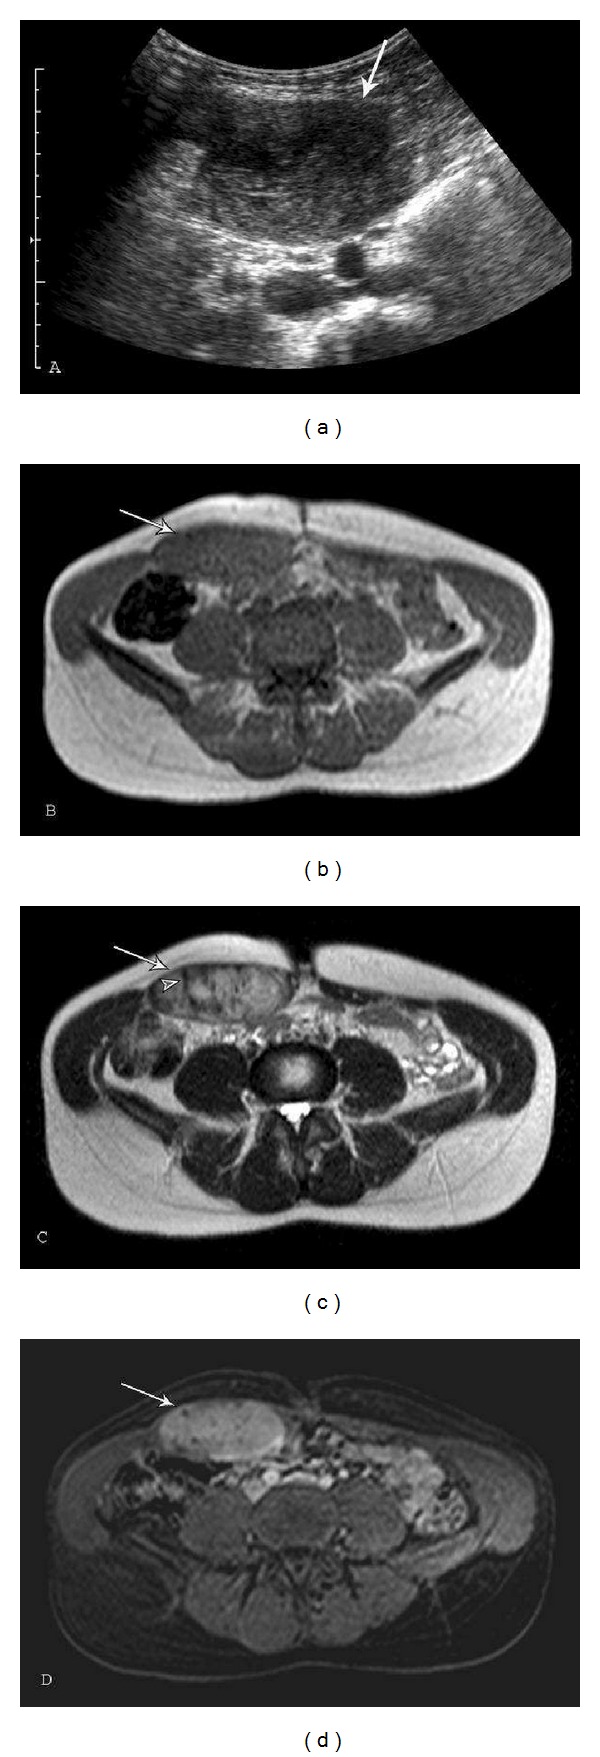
Abdominal fibromatosis of the right rectus abdominis muscle: a 35-year-old female with right rectus abdominis fibromatosis. The lesion continued to grow despite discontinuing oral contraceptive pills and taking ibuprofen. (a) Transverse ultrasound image demonstrates a well-defined, heterogeneous hypoechoic mass. (b) Axial T1- (TR148/TE4.6) and T2-weighted (TR441.2/TE100) sequences reveal a heterogeneous lesion (arrows) with T1 signal similar to skeletal muscle and intermediate to high T2 signal. Low-signal bands (arrowhead) are best evaluated on the (c) T2-weighted image. (d) Axial dynamic thrive (TR4.27/TE2.06) postcontrast fat suppression sequence reveals moderate and diffuse enhancement (arrow) of this lesion with high cellularity and scattered nonenhancing foci corresponding to the collagenized bands.
